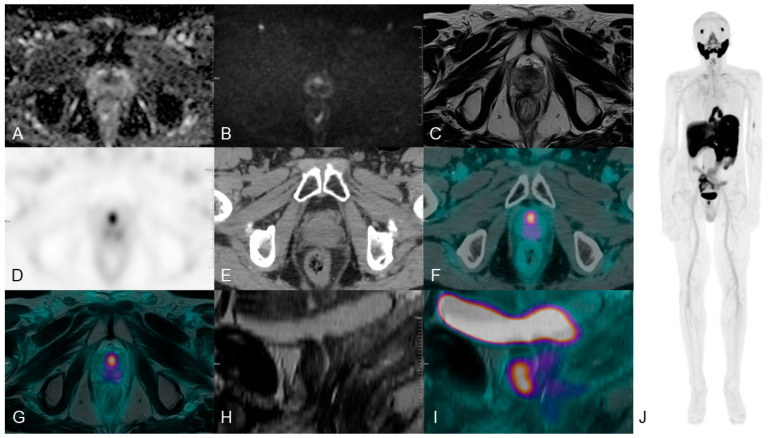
MRI and PSMA-PET/CT in intra-prostatic relapse evaluation. Long Axial Field-of-View (LAFOV) Total-Body PET/CT (194 cm uEXPLORER, United Imaging) with 18F-Piflufolastat (3.35 MBq/kg) in a patient with PSA 3.98 ng/mL, Gleason 6 (3 + 3), post-radiotherapy. Panels: (A) ADC; (B) high b-value DWI; (C) T2-weighted MRI; (D) attenuation-corrected PSMA-PET; (E) low-dose CT; (F) fused PET/CT; (G) axial PET/T2 fusion; (H) sagittal T2; (I) sagittal PET/T2 fusion; (J) PET MIP. PET tracer distribution is visualized using the ‘Warm-Metal’ linear color scale. Image reconstruction and post-processing were performed usingUnited Imaging Healthcare PET/CT and CT Image Post-processing Software (uWS-MI:R002.32.0.1749877; uWS-CT:F006.34.0.1749877), where higher metabolic activity or tracer avidity is represented by the transition from cooler tones (black/green) to warmer tones (orange/white). MRI shows a focal lesion with diffusion restriction and T2 hypointensity, matching PET uptake along the anterior midline. MRI clarifies urethral position, confirming true intraprostatic relapse. Complementary MRI-PET improves lesion characterization. Images courtesy of Nuclear Medicine Centre, San Pietro Fatebenefratelli Hospital, Rome, Italy.
